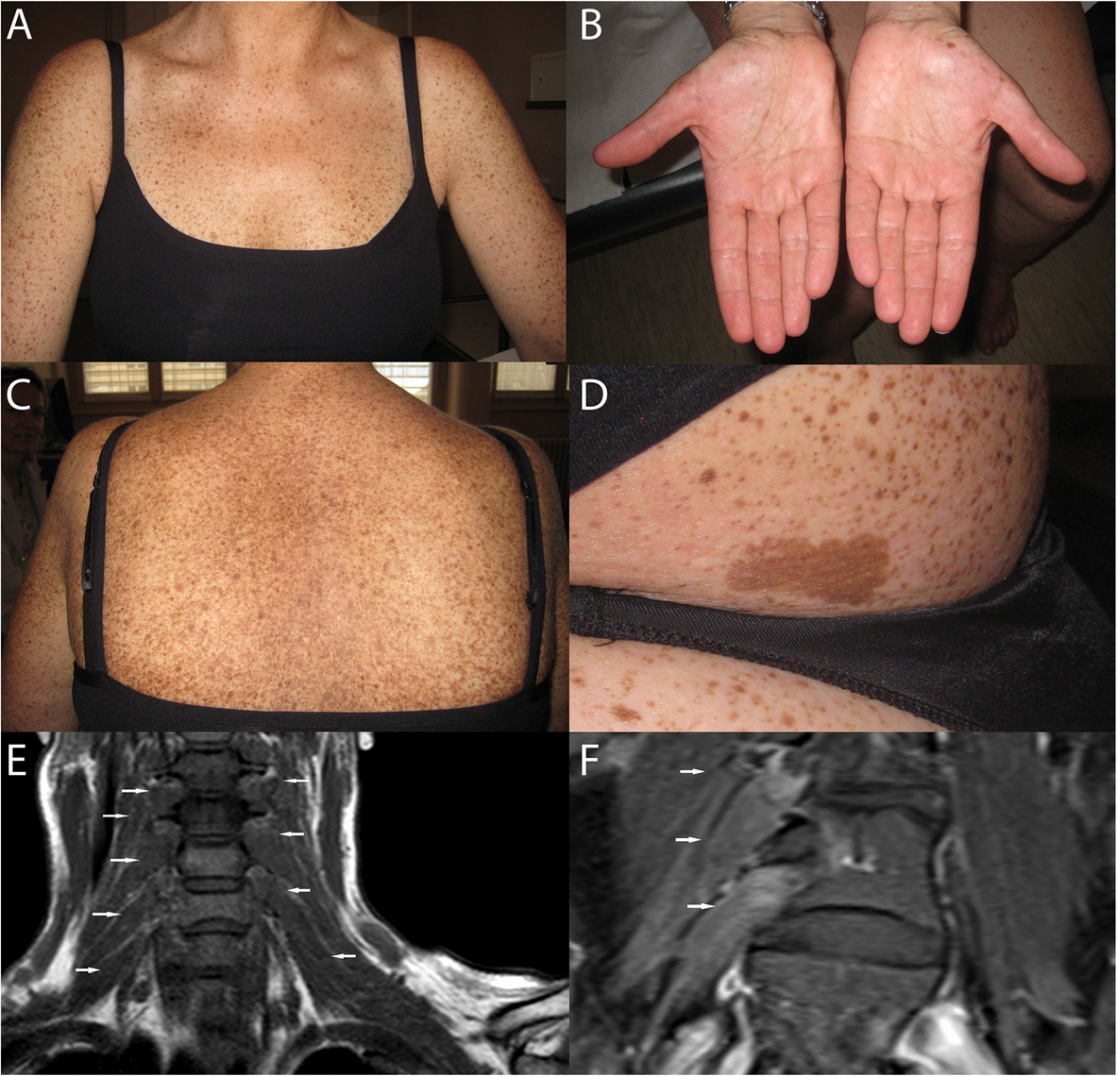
Clinical and MRI features of LEOPARD syndrome presenting with hypertrophic plexi and neuropathic pain. Patient with LEOPARD syndrome showing multiple cutaneous lentigines (A and C: trunk, B: palms) and café-au-lait spots, on the upper buttock (D). Spinal MRI showing hyperthrophy of the cervico-brachial (E) and lumbo-sacral (F) plexi.
